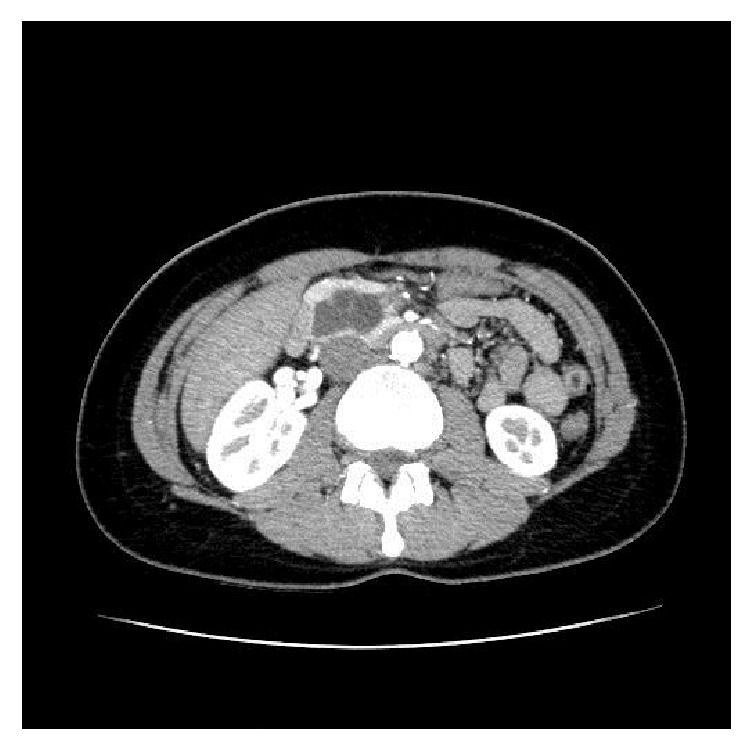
Abdominal CT scan showing a large cystic lesion in the head of the pancreas.
